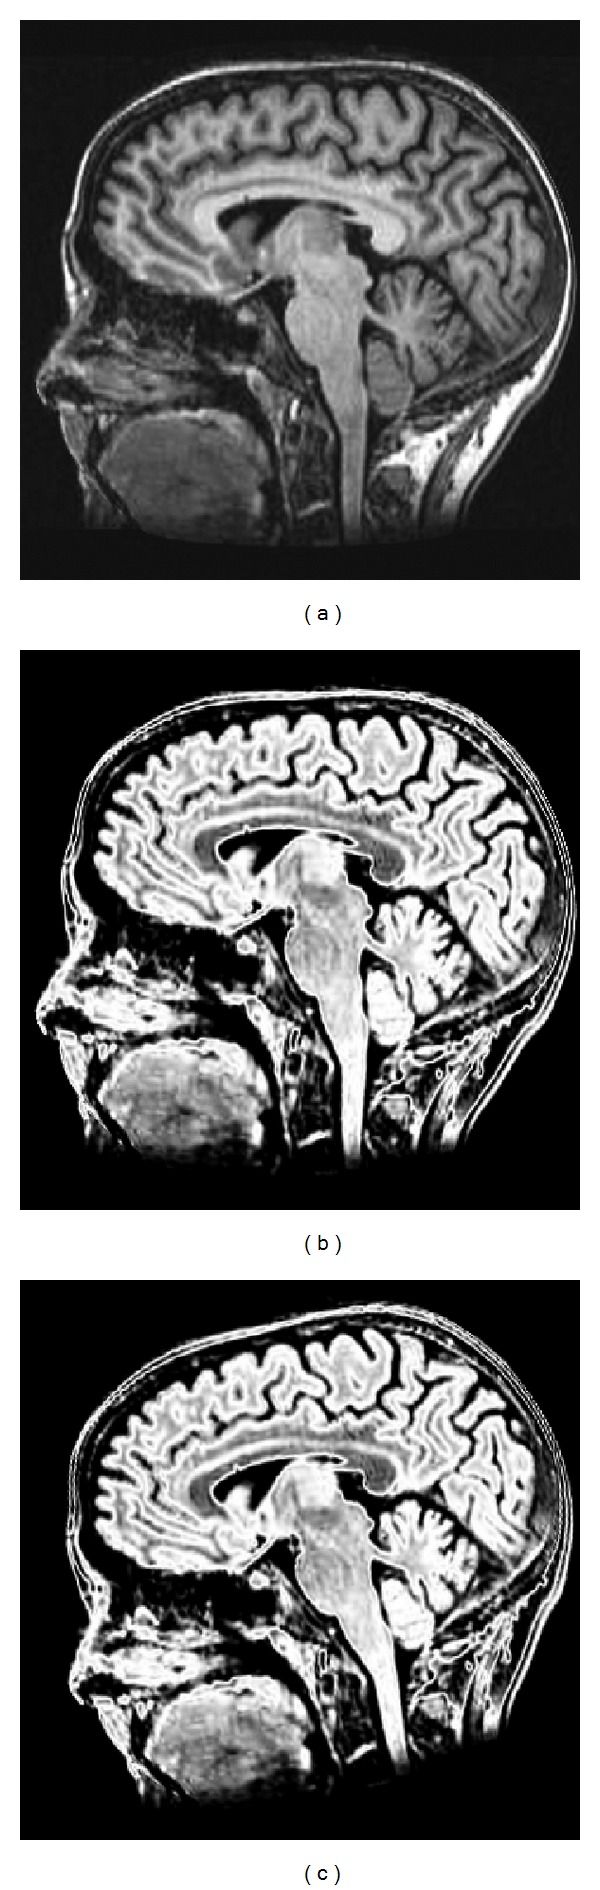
Test MR Brain images. (a) T1 MRI. (b) T2 MRI. (c) Rotate T2 MRI by 11.46°.
